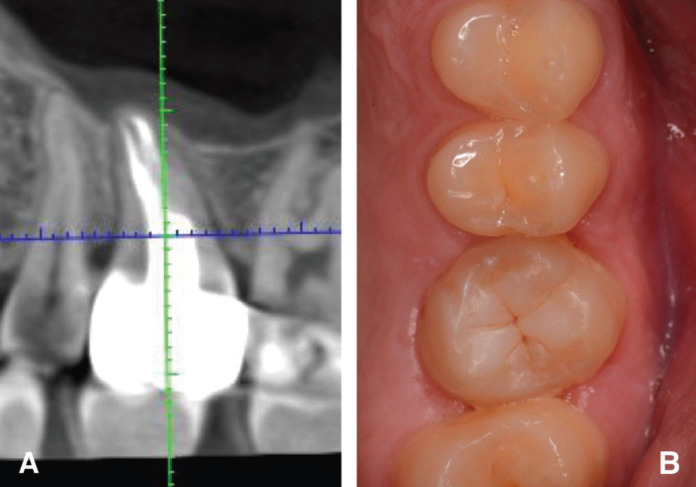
A. CBCT scans after 30 months postsurgery. B. Condition of soft tissue, 30 months after surgery.
